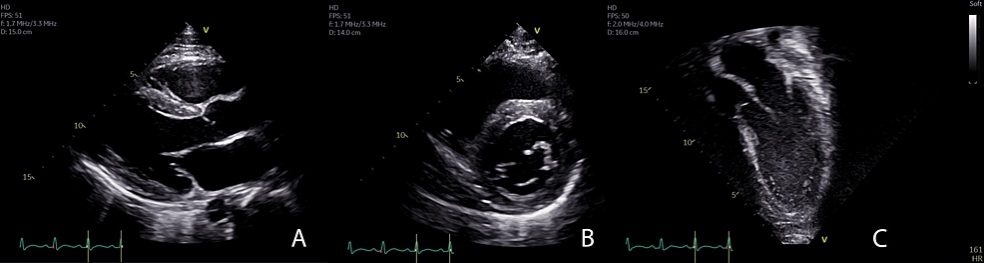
Echocardiogram at initial presentation Transthoracic echocardiography of the parasternal long axis (A), parasternal short axis (B), and apical three chamber view (C) were obtained on day one of hospitalization prior to initiation of treatment for MIS-C. There was no hypertrophy of the left ventricle.
